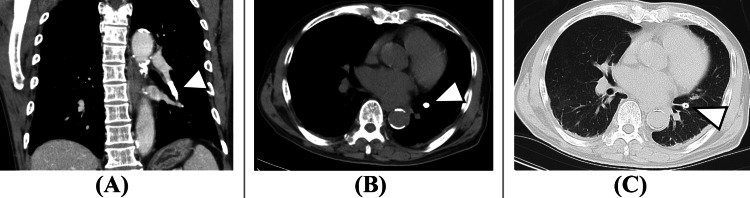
Immediate postoperative chest CT. (A) Contrast-enhanced coronal view. (B) Axial view (soft tissue window). (C) Axial view (lung window). A foreign body consistent with Nelaton cover embolism is visible in the left pulmonary artery (white arrowheads, A-C).
